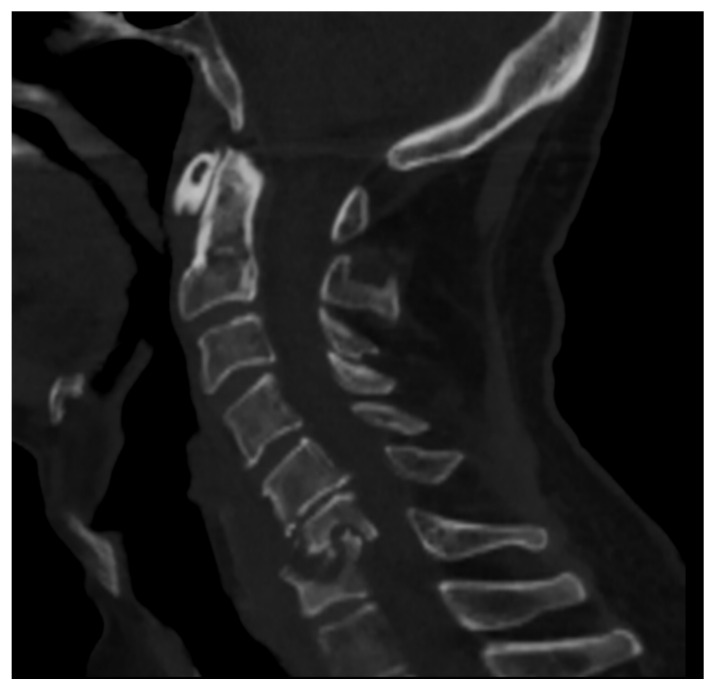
CT of the cervical spine (sagittal reconstruction) of a middle-aged man (HIV+) affected by spondylodiscitis in the C6–C7 tract of the cervical spine complicated by myelopathy.
